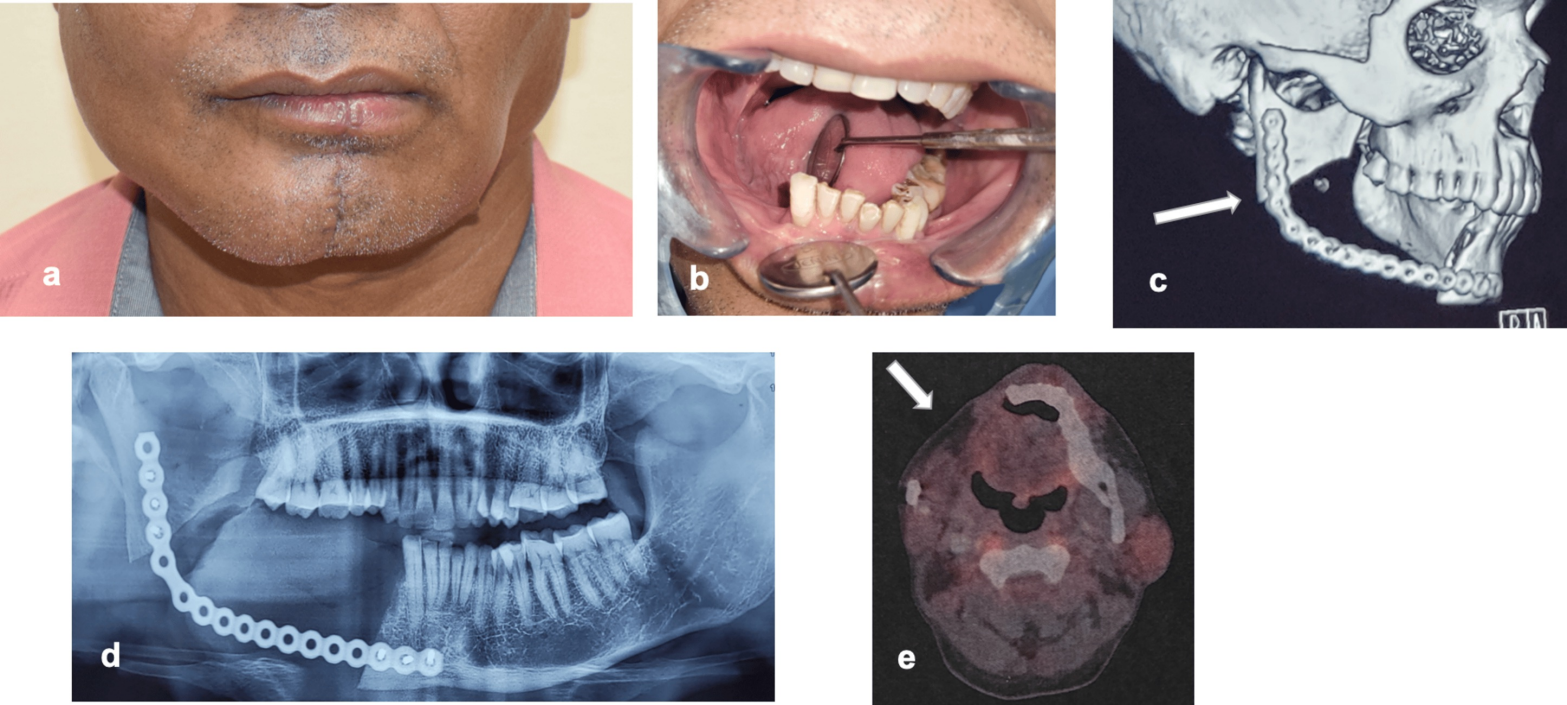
a: Postoperative extraoral image. b: Postoperative intraoral image. c: Postoperative 3D CT image. d: Postoperative OPG. e: Postoperative PET scan OPG: Orthopantomogram
